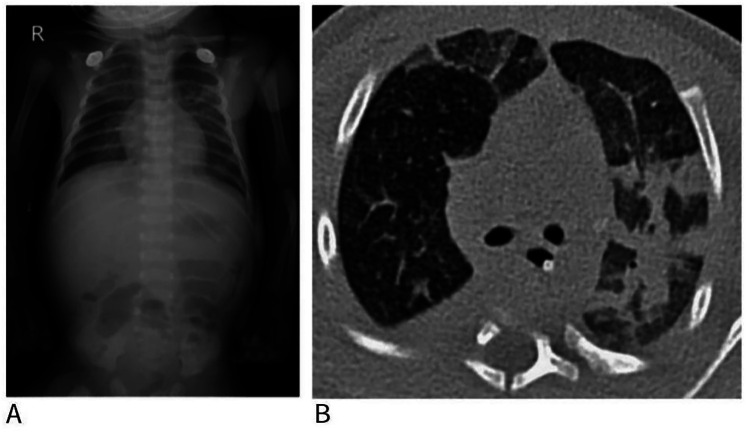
Chest radiograph and CT revealed bilateral multifocal infiltrates. (A) Chest radiograph obtained at admission; (B) CT scans taken on the 11th day of hospitalization.
